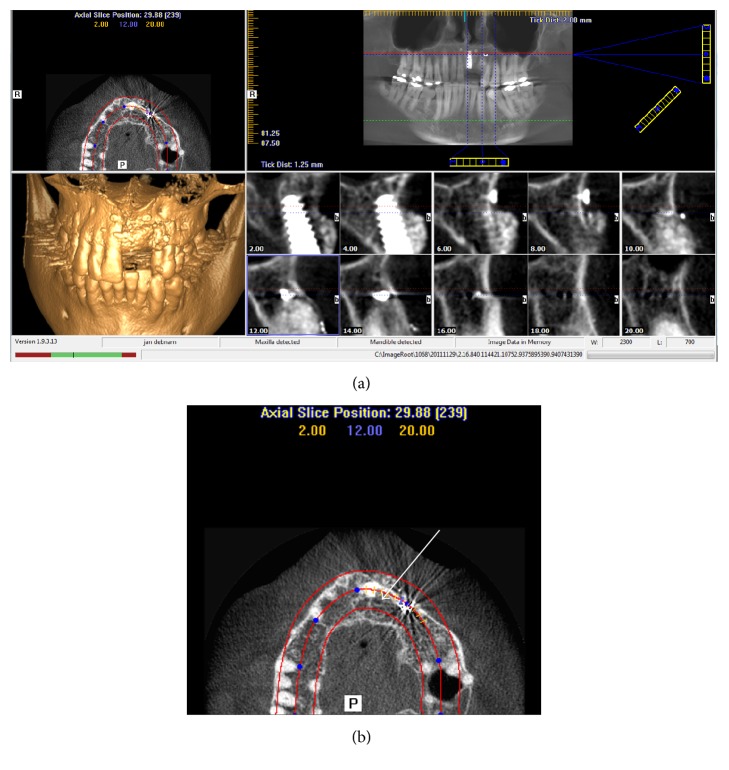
(a) Postoperative CBCT (iCAT) imagery taken at implant clinic to investigate for pathology at osteotomy site 21. The cross-sectional views show that the implant has penetrated the anterolateral wall of the nasopalatine duct. Additionally, the xenograft granules are shown as remaining separate from the alveolus buccal to the implant. (b) Magnified axial image from (a) again showing that a well-demarcated canal is present between the apex of tooth 23 and the nasopalatine duct and palatally to the newly placed implant, white arrow.
